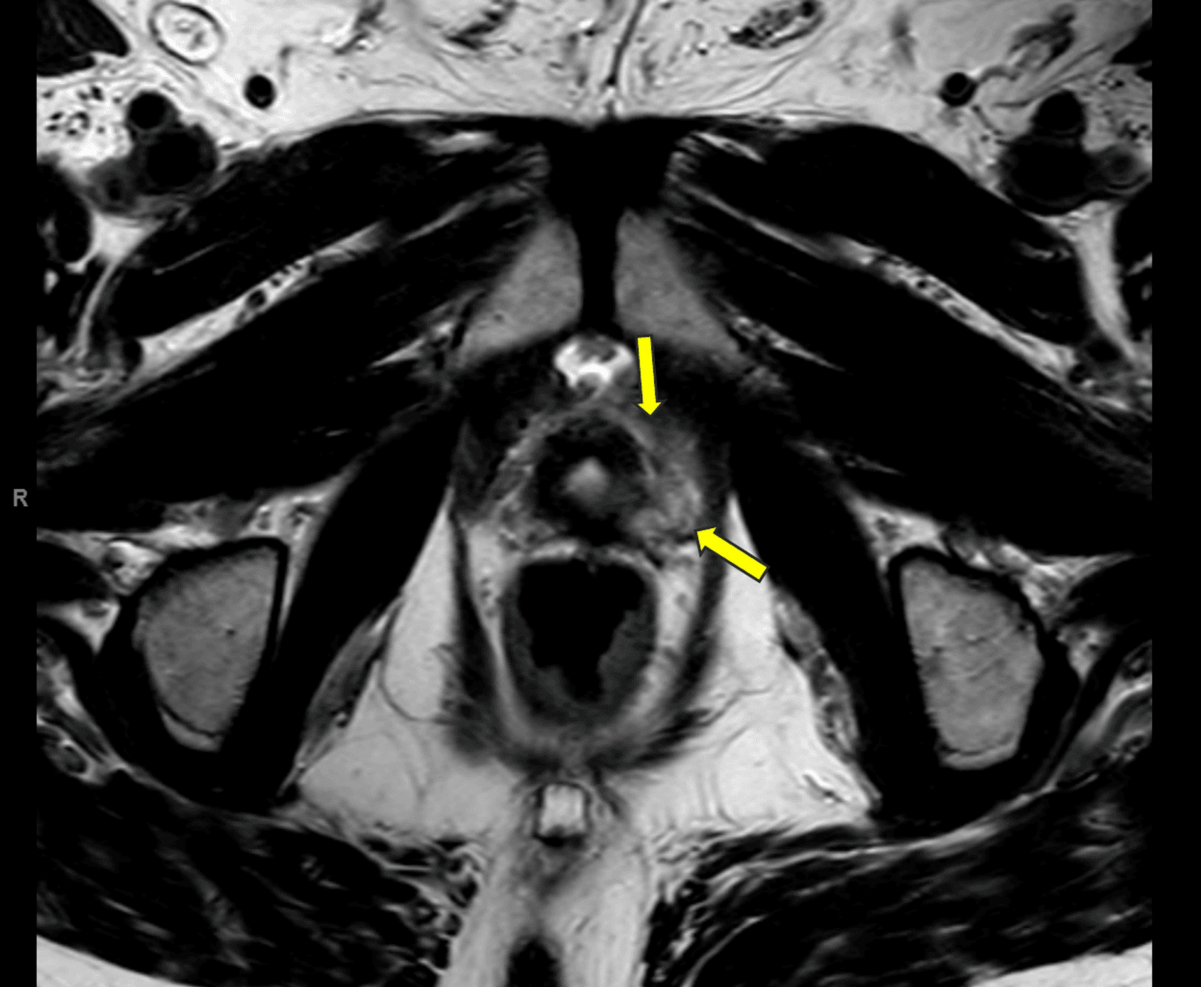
Axial T2 TSE MRI displaying loss of T2 hypointense signal in the left lateral aspect of the bladder neck The yellow arrows point to the area of recurrent disease adjacent to the bladder neck. TSE: turbo spin echo, MRI: magnetic resonance imaging
